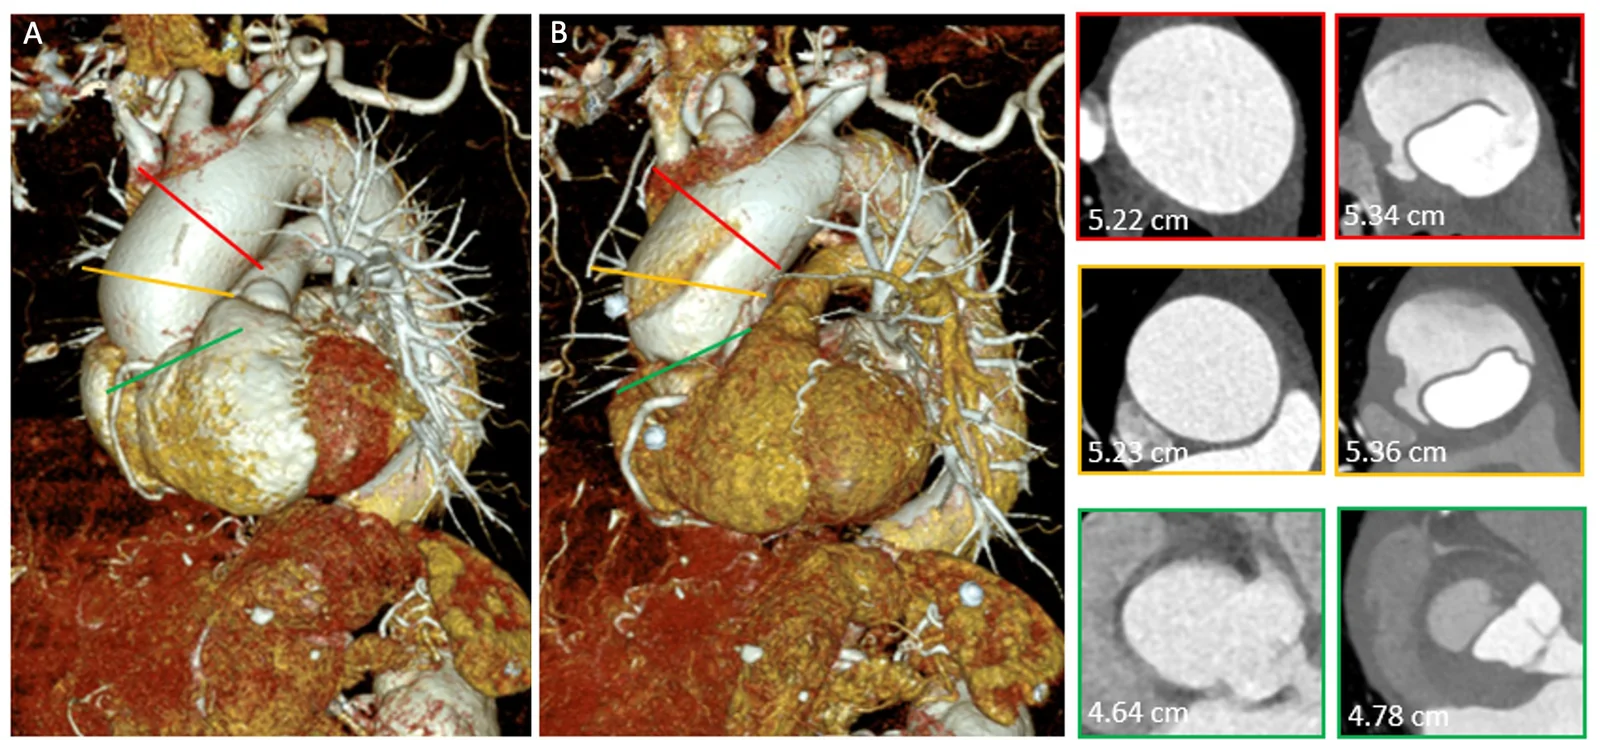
Panel A and B: Pre- and post-acute aortic dissection computed tomography angiography (CTA) imaging, revealing the morphological and geometrical changes at different segmental aortic planes during the dissecting process (aortic root, green line; mid-ascending aorta, yellow line; transition from the distal ascending aorta to the proximal aortic arch, red line).
